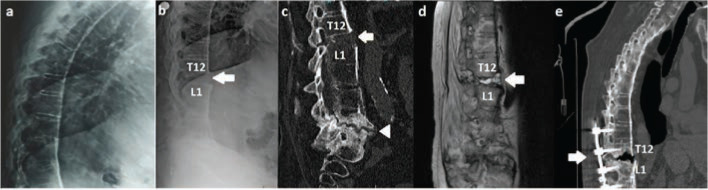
(a) Lateral X-ray of thoracolumbar spine showed bamboo spine. (b) Lateral X-ray of thoracolumbar spine showed a fracture across the disc and the posterior elements at the T12-L1 level. (c) A sagittal-reformatted CT scan of thoracolumbar spine confirmed a horizontally oriented three-column fracture at T12 and L1 level (arrow). An old, inadequately healed chalk stick fracture with sclerotic margins was also seen at L4-L5 level (arrow head). (d) Sagittal proton-density MR image showed altered signal intensity of the disc, the vertebral bodies, and the posterior elements at the T12-L1 level. (e) A postoperative sagittal-reformatted CT scan of thoracolumbar spine showed extensive fixation with pedicle screws and connective rods.
